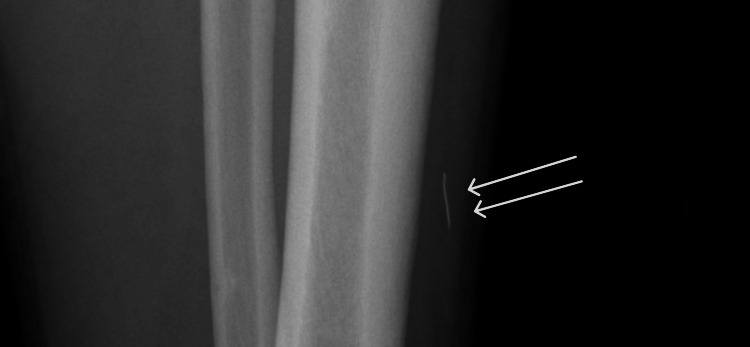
Left leg X-ray. The white arrows point toward the foreign body object.
